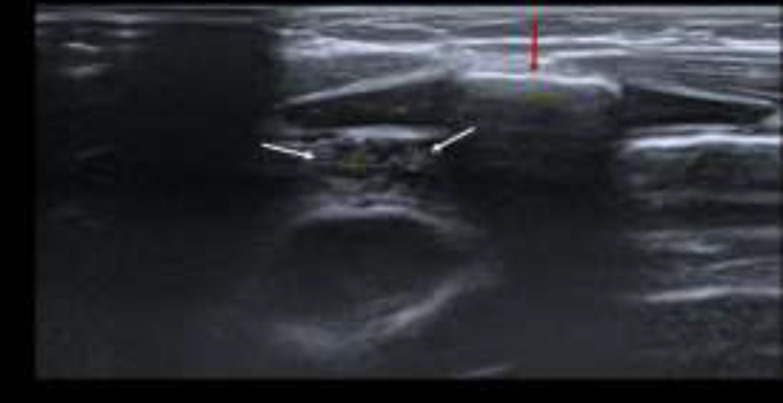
Ultrasound scan shows normal thymus (as shown by white arrows) in the thoracic inlet deep to sternum (red arrow) and no movement of the normal thymus appreciated when patient was crying
